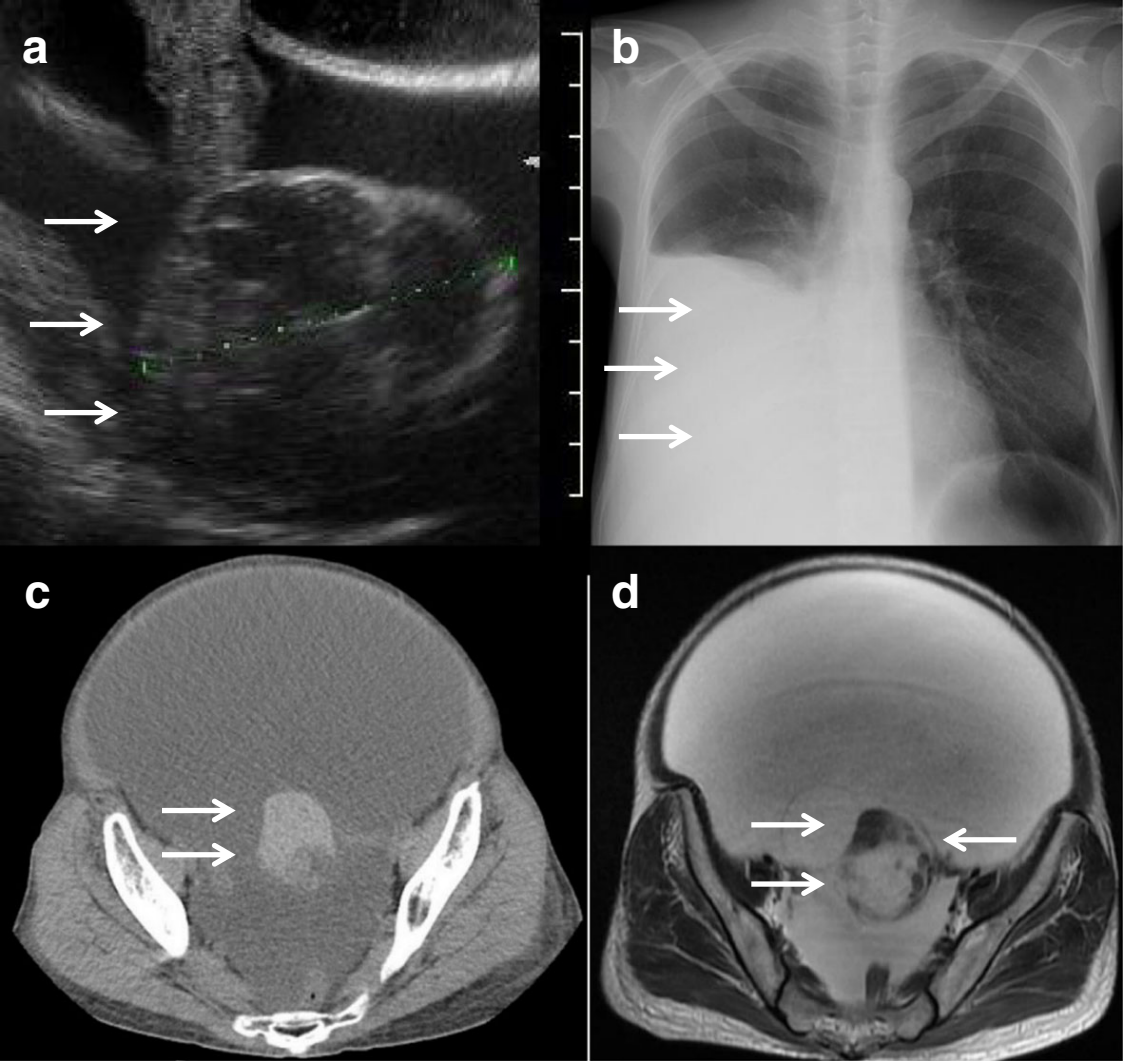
Transvaginal US showing marked ascites and a large solid and cystic mass with a diameter of 8 cm in the left ovary (arrows) (a). Chest X-ray showing a massive right-sided pleural effusion (arrows) (b). CT scans showing a solid component of the tumor with a higher attenuation lesion (arrows) (c). T2-weighted MRI showing the cystic lesions with various intensities separated by the septa (arrows) (d)
